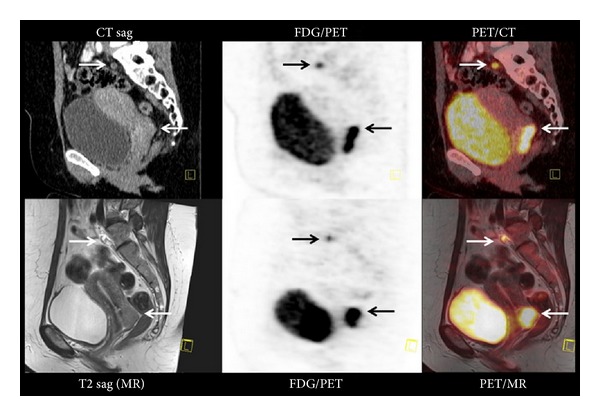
31-year-old female patient with cervical cancer had an ^18^F FDG-PET/CT and PET/MRI examination for restaging following radiotherapy. PET/CT showed the primary tumour in the cervix behind the urine bladder and a lymph node in the pelvis, both indicated with arrows. PET/MR exhibited the same findings but with a more precise definition of the primary tumor (adopted from Kjær et al. [55]).
